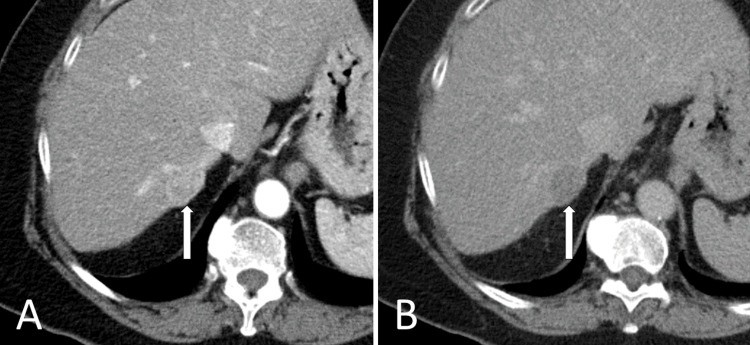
Liver protocol CT demonstrating LIRAD V lesion in segment 7 (A) Arterial phase, arterially enhancing lesion in segment 7; (B) Delayed phase demonstrates washout LI-RADS: Liver Imaging Reporting and Data System
